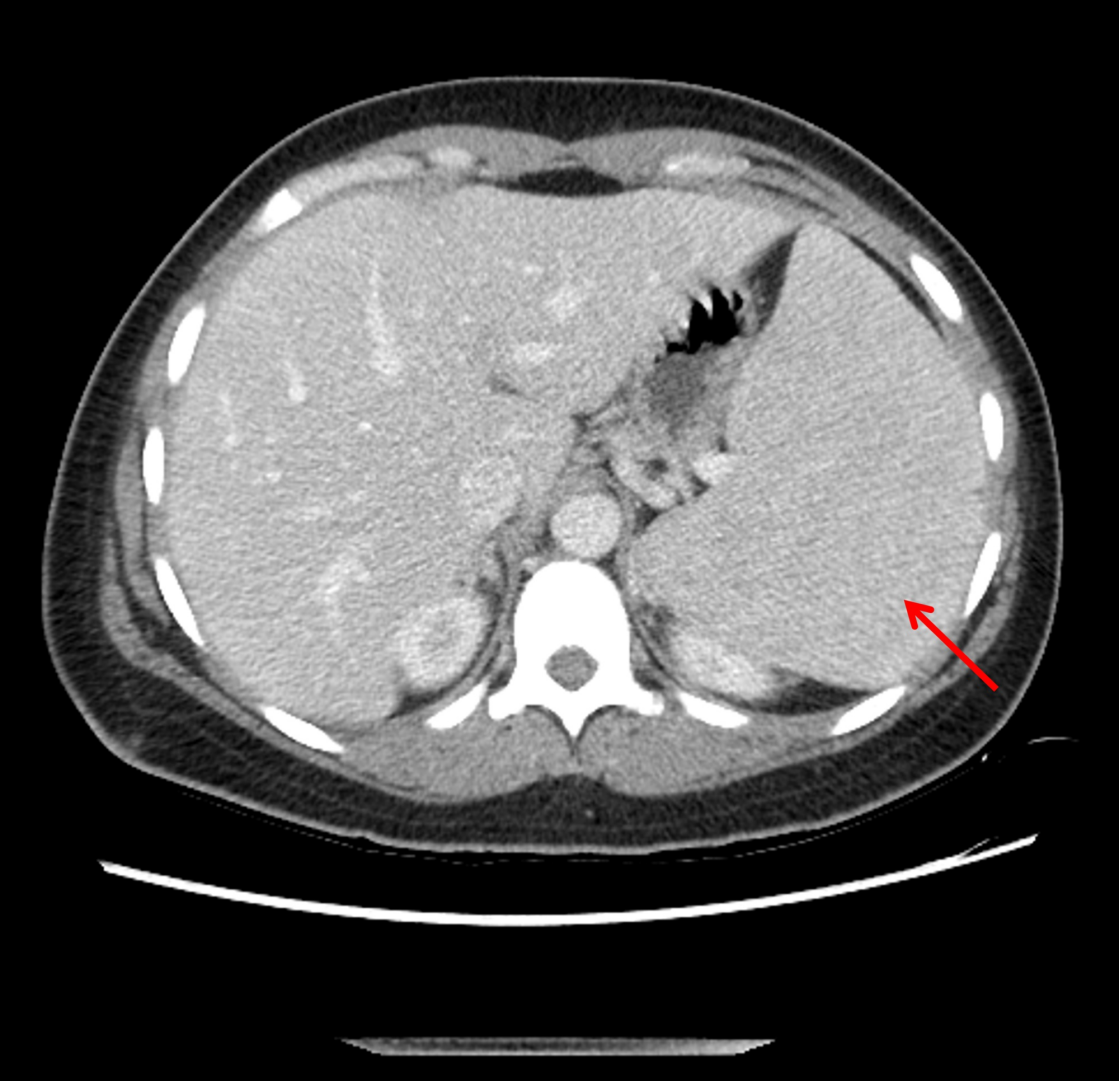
Computed tomography scan of the abdomen demonstrating splenomegaly (red arrow)
